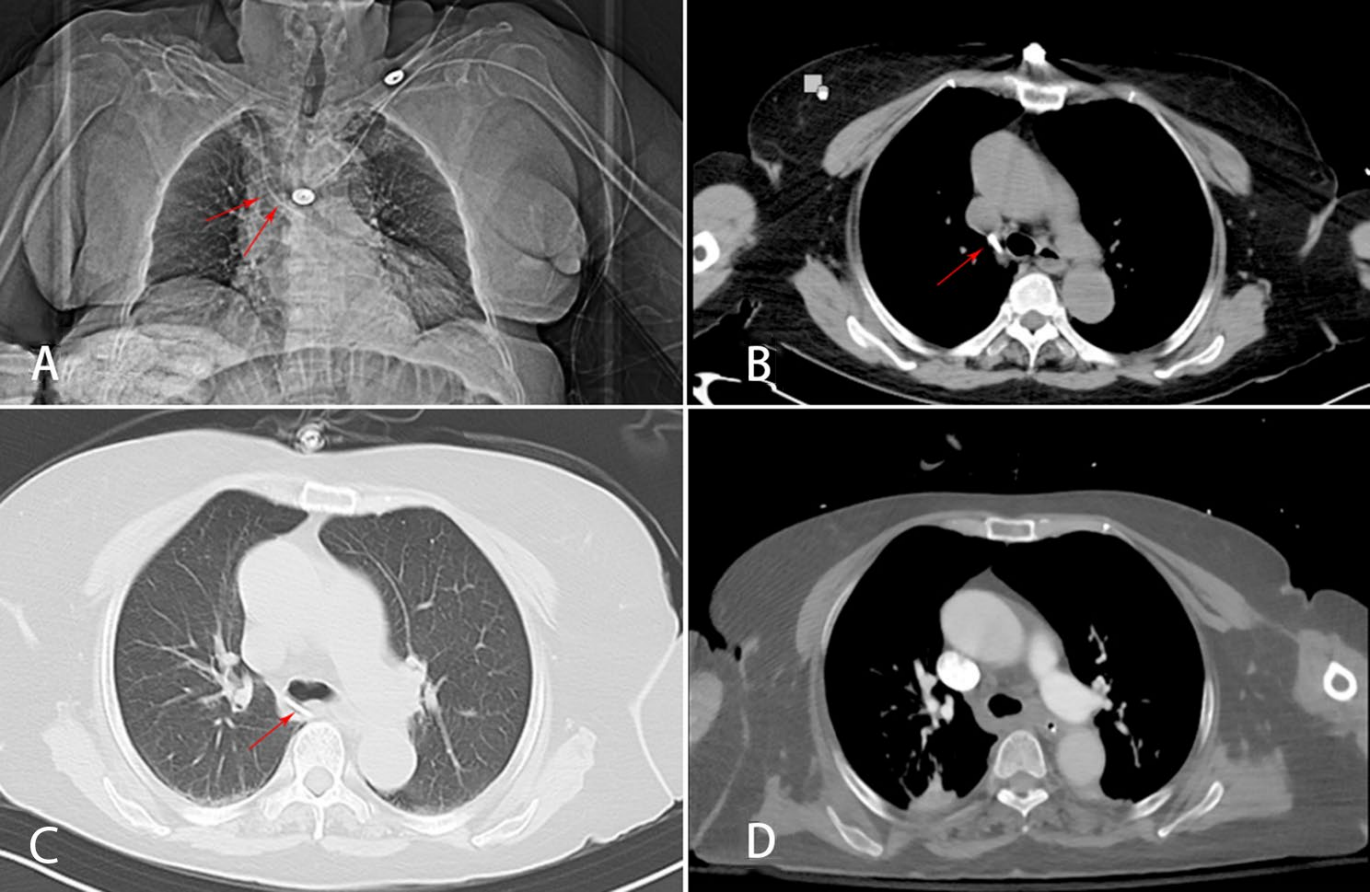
(A) Chest radiograph showing a central venous catheter inserted via the right subclavian vein and the catheter kinking at the right tracheobronchial angle (red arrows); (B) Axial slice from the chest CT scan shows the catheter tip projecting from the posterior wall of the superior vena cava (red arrow); (C) The CVC deviated anteriorly towards the thoracic vertebrae (red arrow); (D) Post-operative contrast-enhanced chest CT revealed no pleural effusion or mediastinal fluid accumulation
